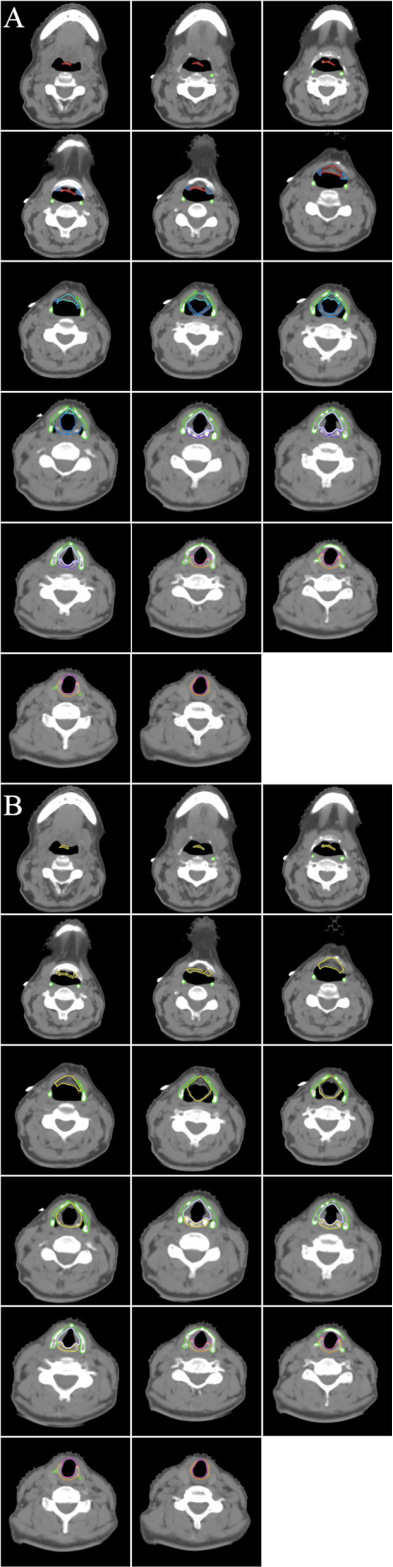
Atlas of the larynx and its substructures on consecutive axial computed tomography (CT) slices: the thyroid cartilage is depicted in green, the cricoid cartilage in orange, the arytenoid cartilages in purple, the suprahyoid epiglottis in red, the infrahyoid epiglottis in cyan, the aryepiglottic fold/false vocal folds in blue, the supraglottic larynx in yellow, the glottic larynx in lavender, and the subglottic larynx in magenta. (A) Individual substructures of the larynx. (B) Major divisions of the larynx.
